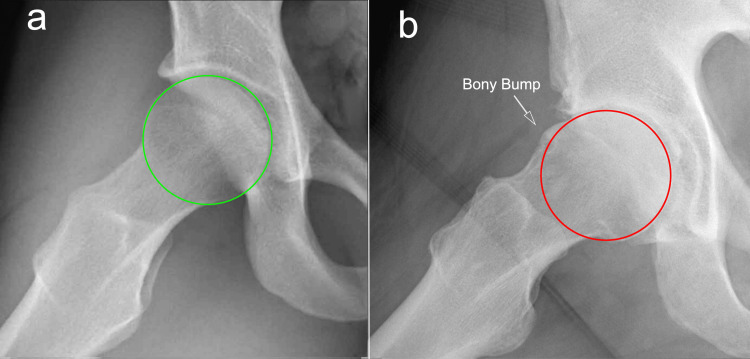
Dunn view radiographs of hip a) Normal hip joint; b) hip joint showing a clear anterior head-neck deformity, defined as a bony bump. Image credit: Author, Saverio Colonna
